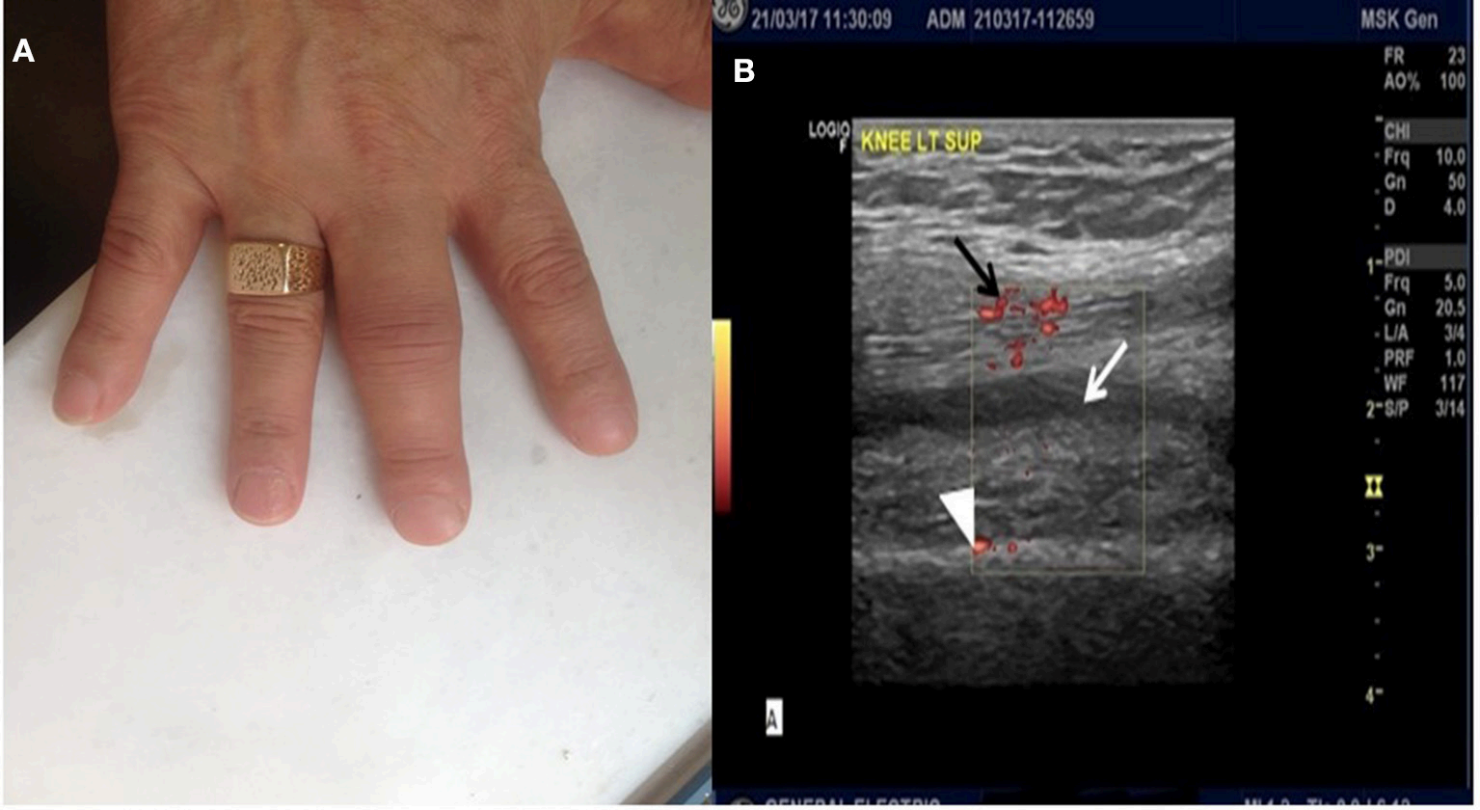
(A) Arthritis of interphalangeal joints of the hands. (B) Ultrasound image of the left knee. Note the presence of synovial fluid (*white arrow*), marked synovial hypertrophy (*white arrowhead*) and presence of Power Doppler signal (*black arrow*), all indicative of active synovial inflammation.
